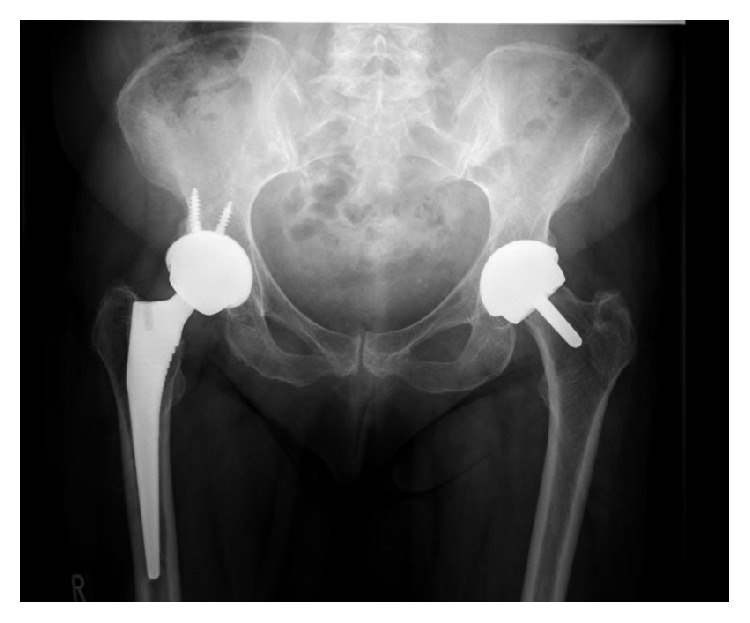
Anteroposterior X-ray pelvis showing right THR and left hip resurfacing.
